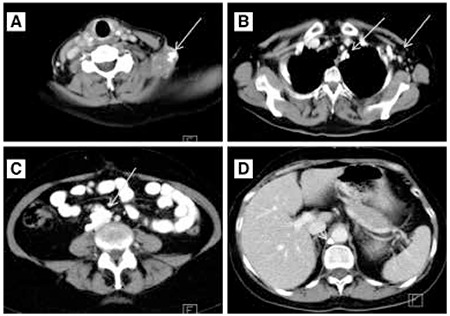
After 2 cycles of chemotherapy, the patient underwent computed tomography for evaluation of treatment response. Size reduction and presence of further calcification in the described lymph nodes were noted. Some of the lymph nodes were completely replaced by calcium deposits (A, B, C, D) (arrows)
